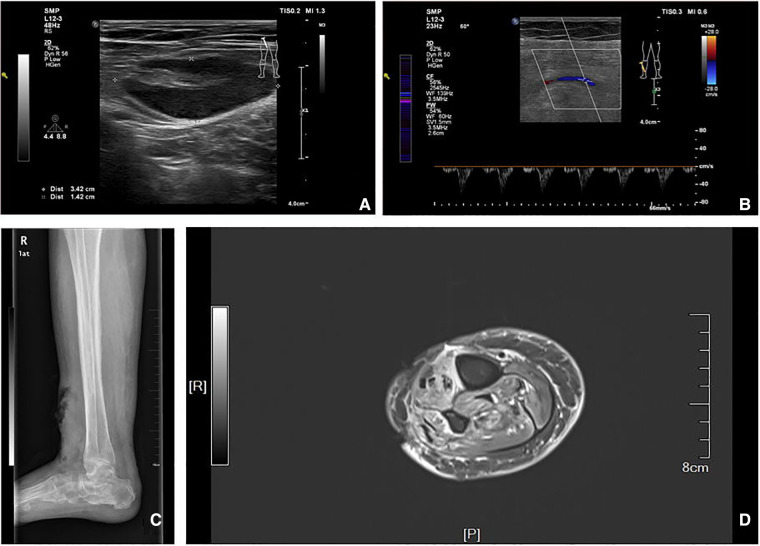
(A,B) Arteriovenous color ultrasound images of the lower limb. (C) Lateral view of the ankle. (D) MRI image of the limb.
